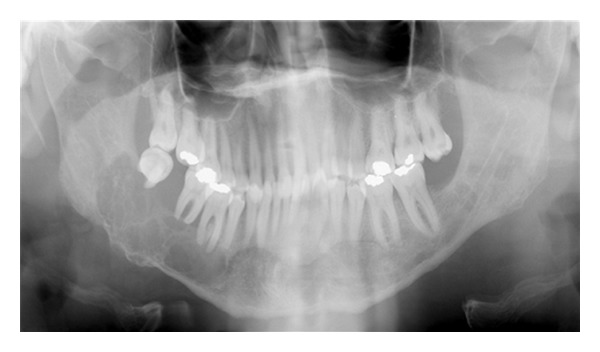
Panoramic radiography showing a multilocular radiolucency of the right mandible.
